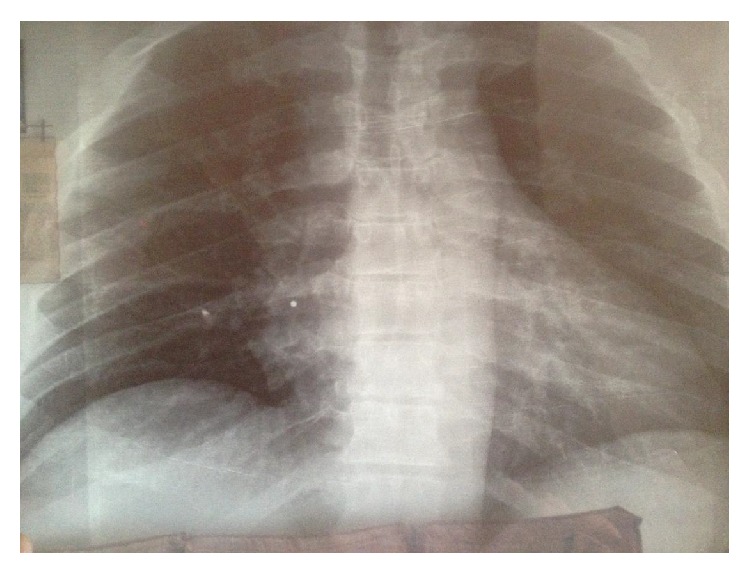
X-ray of the thorax. A small-sized round object of metal density (pellet) lies in the proximity of the right atrium.
